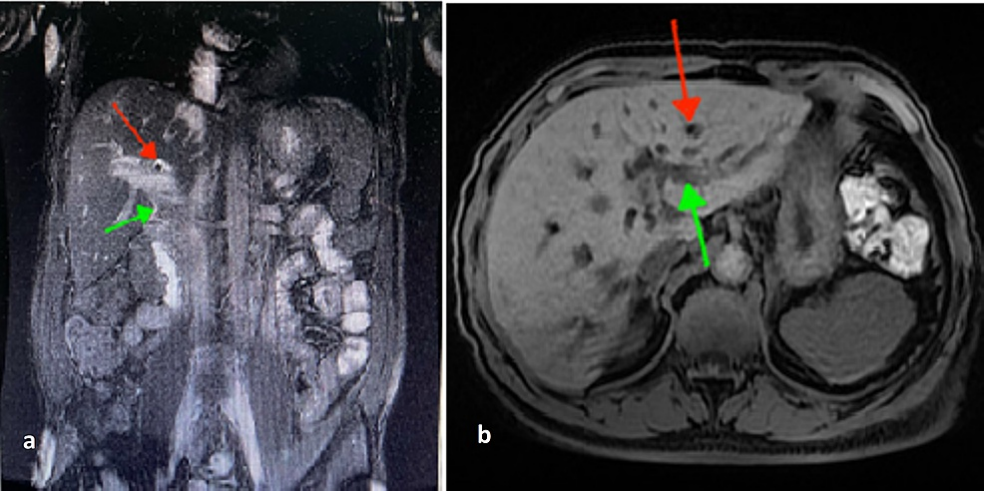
A 70-years-old patient with cholangitis: MRI with coronal T2 (a) and axial T1 (b) slice showing lithiasis in the absence of signal from the intrahepatic bile ducts (red arrow) with segmental dilations (green arrow). MRI: magnetic resonance imaging.
